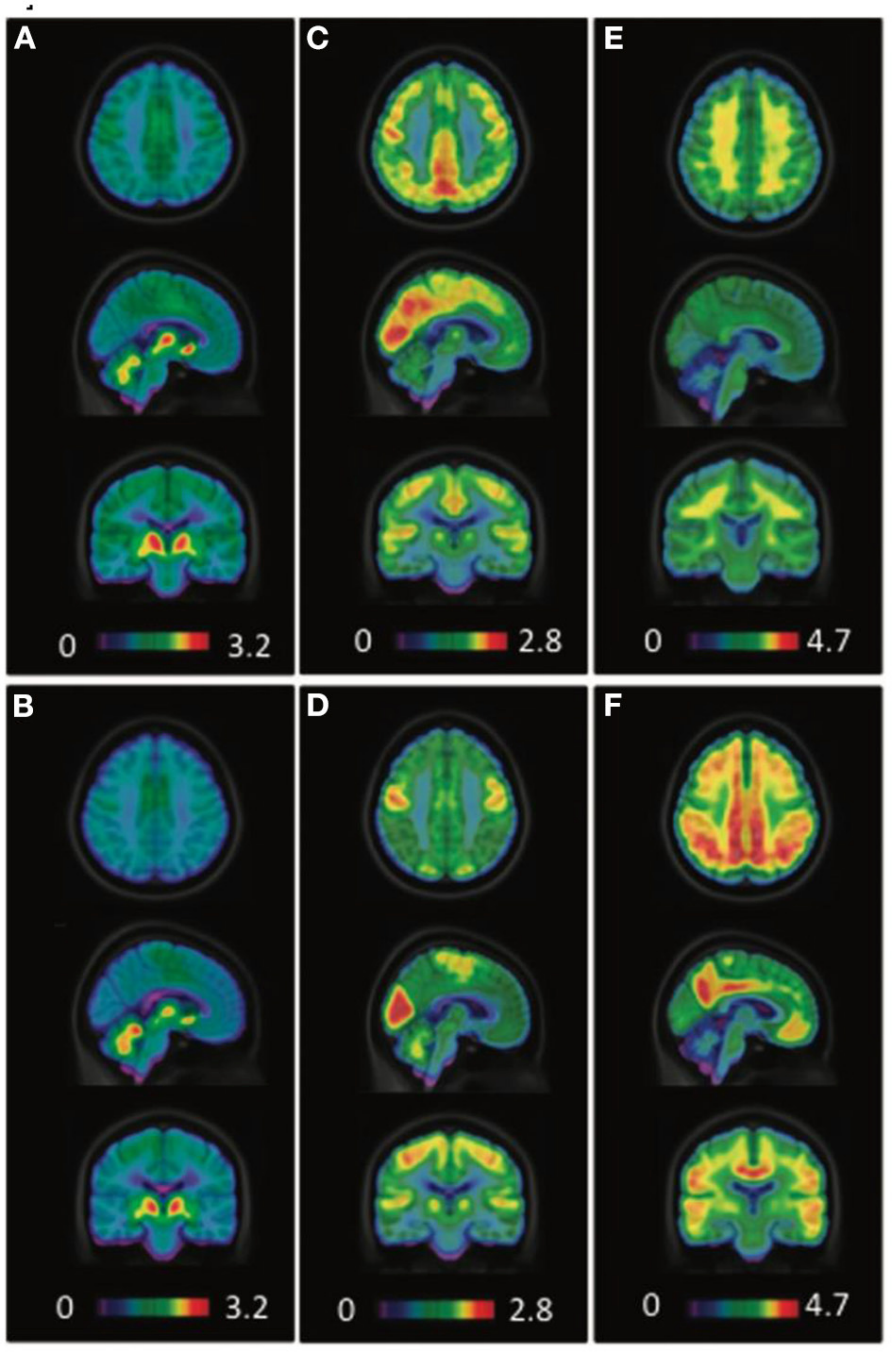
Averaged [^18^F]FEOBV VAChT PET (A,B), [^18^F]FDG PET (C,D), and [^18^F]NAV4694 Aβ PET (E,F) images of healthy controls (A,C,E) and AD patients (B,D,F). PET images in control subjects revealed the greatest [^18^F]FEOBV uptake in brain areas known to be the most innervated by the cholinergic systems, including the striatum, thalamus, cerebral cortex as a whole, hippocampal area and cerebellum (A). In AD patients, PET imaging revealed a significant reduction of [^18^F]FEOBV uptake in comparison with control subjects (B). Distinct cerebral hypometabolism (D) and amyloid deposition (F) were seen in the AD patients, as compared to control subjects (C,E). Courtesy from Aghourian et al. (2017).
